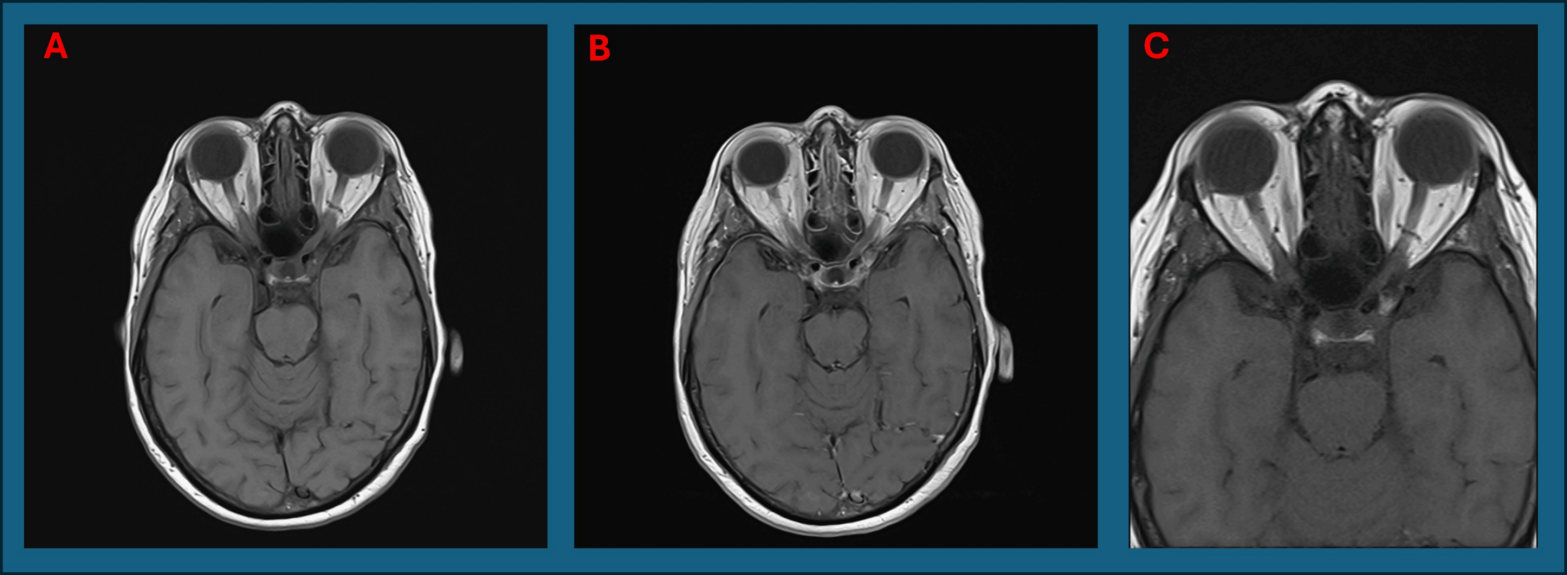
A: Normal axial T1 MRI head sequence pre-contrast from second presentation. B: Normal axial T1 MRI head sequence post-contrast from second presentation. C: Normal Axial T1 MRI Head sequence no contrast used on outpatient review after second discharge
